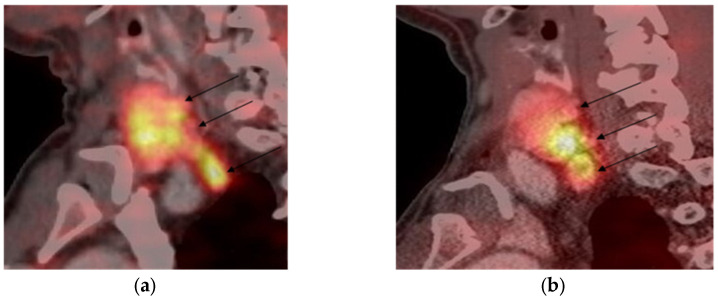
PET/CT-scans: (a) three [68Ga]-DOTA-TATE positive lesions in allocation of right upper (10 × 5 × 7 mm), middle (15 × 10 × 23mm), and lower (14 × 10 × 24 mm) parathyroid glands (arrows); (b) [11C]-choline PET/CT demonstrates all lesions have high [11C]-choline uptake (arrows).
